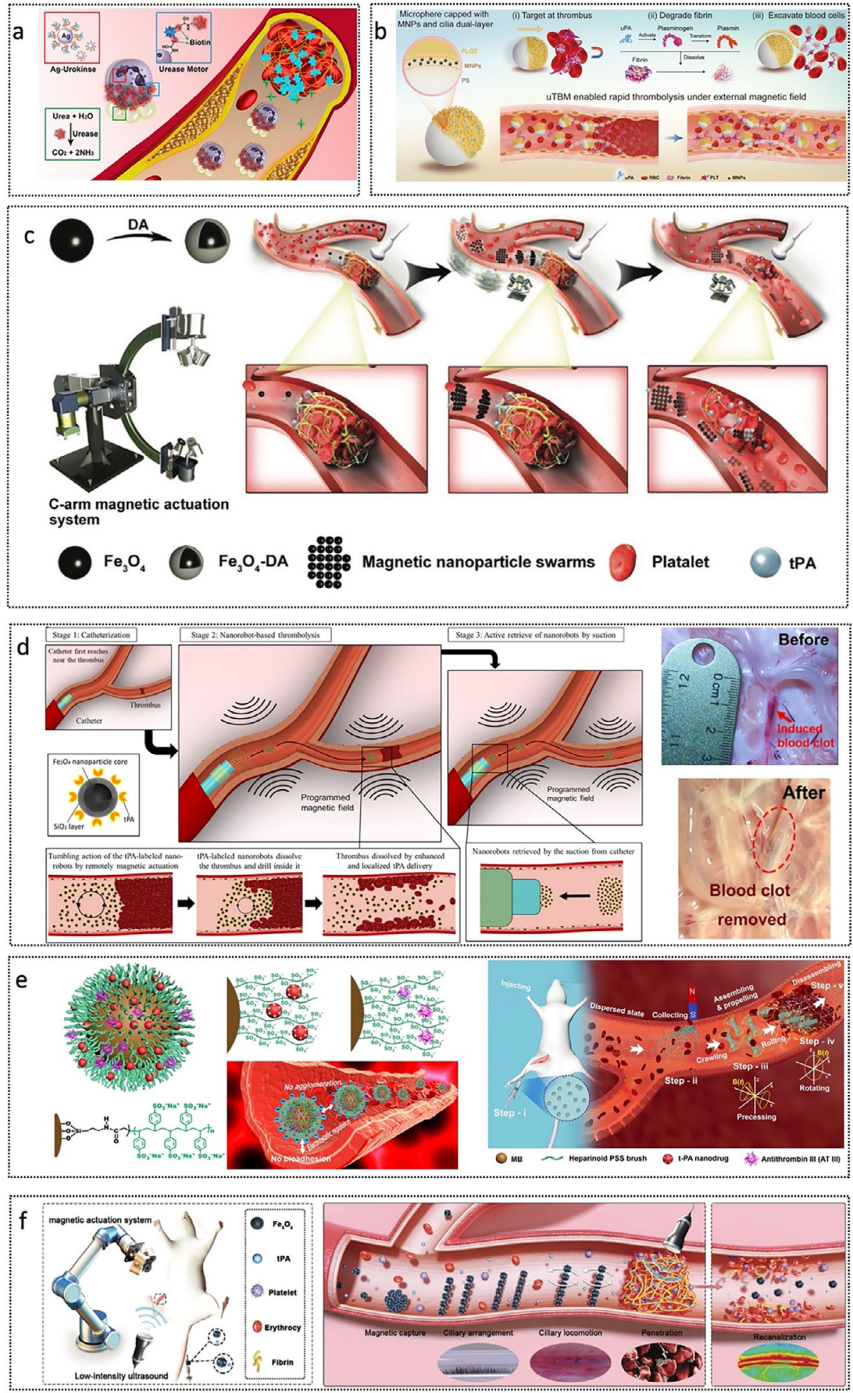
Micro/nanorobots for combating thrombus. a) Enzyme catalysis biomotor engineering of neutrophils. Reproduced with permission from ref. [163] Copyright 2022, American Chemical Society. b) uTBMs enabled rapid thrombolysis under the magnetic field manipulation. Reproduced with permission from ref. [164] Copyright 2025, Wiley‐VCH. c) Nanorobots actuated by a C‐shaped magnetic actuation system for enhanced thrombolysis in vivo. Reproduced with permission from ref. [168] Copyright 2021, Wiley‐VCH. d) tPA‐anchored nanorobots. Reproduced with permission from ref. [165] Copyright 2024, American Association for the Advancement of Science. d) Swarming HPB‐NRs based on MB@PSS NPs. Reproduced with permission from ref. [166] Copyright 2023, American Association for the Advancement of Science. e) Treatment using magnetically actuated colloids and low‐intensity ultrasound. Reproduced with permission from ref. [167] Copyright 2025, Wiley‐VCH.
